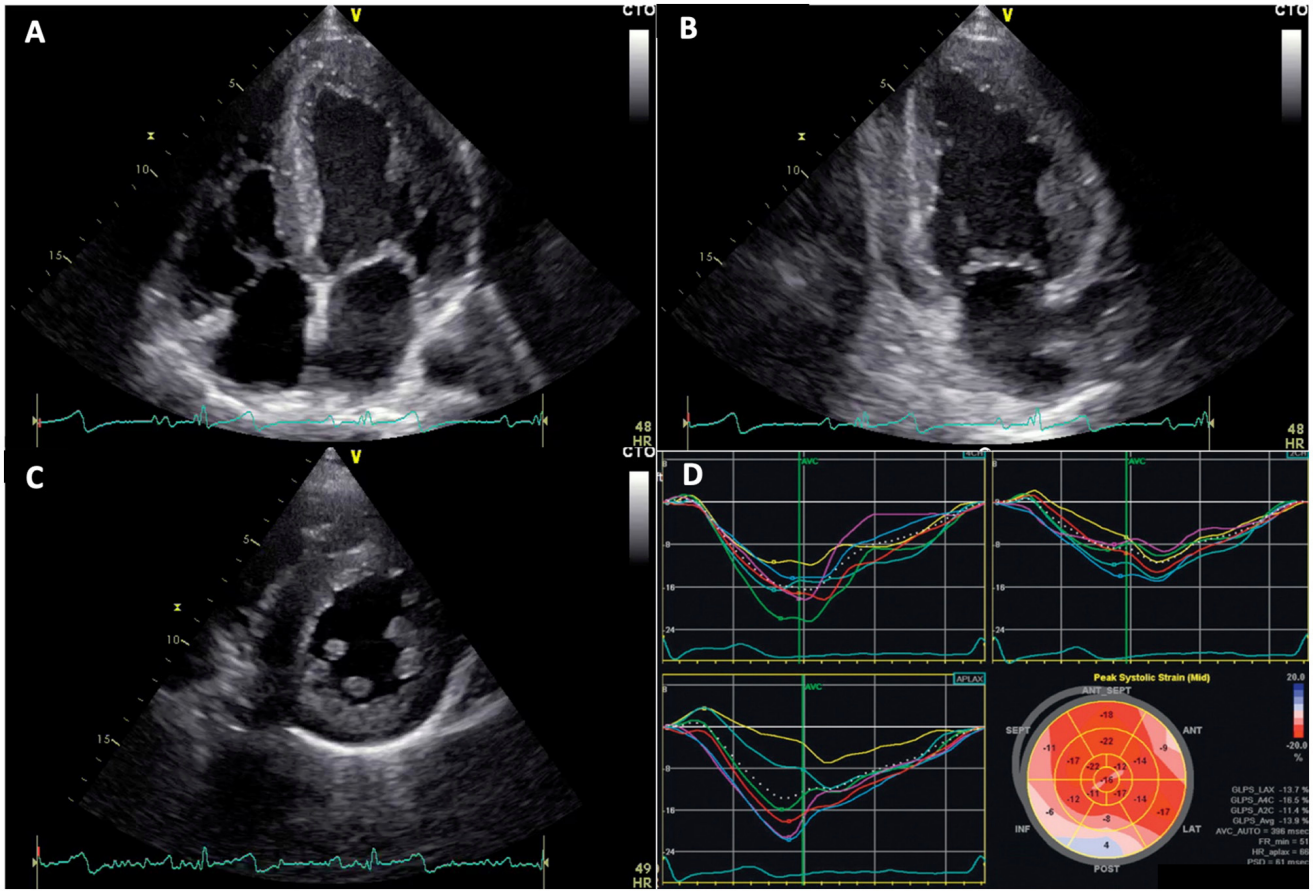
Echocardiographic images of Fabry disease (FD) patients. (A–C) apical 4 chambers, 2 chambers, and parasternal short axis views showing left ventricular concentric hypertrophy typical of FD, respectively. (D) altered global longitudinal strain in patient with FD, note inferolateral segment alterations.
